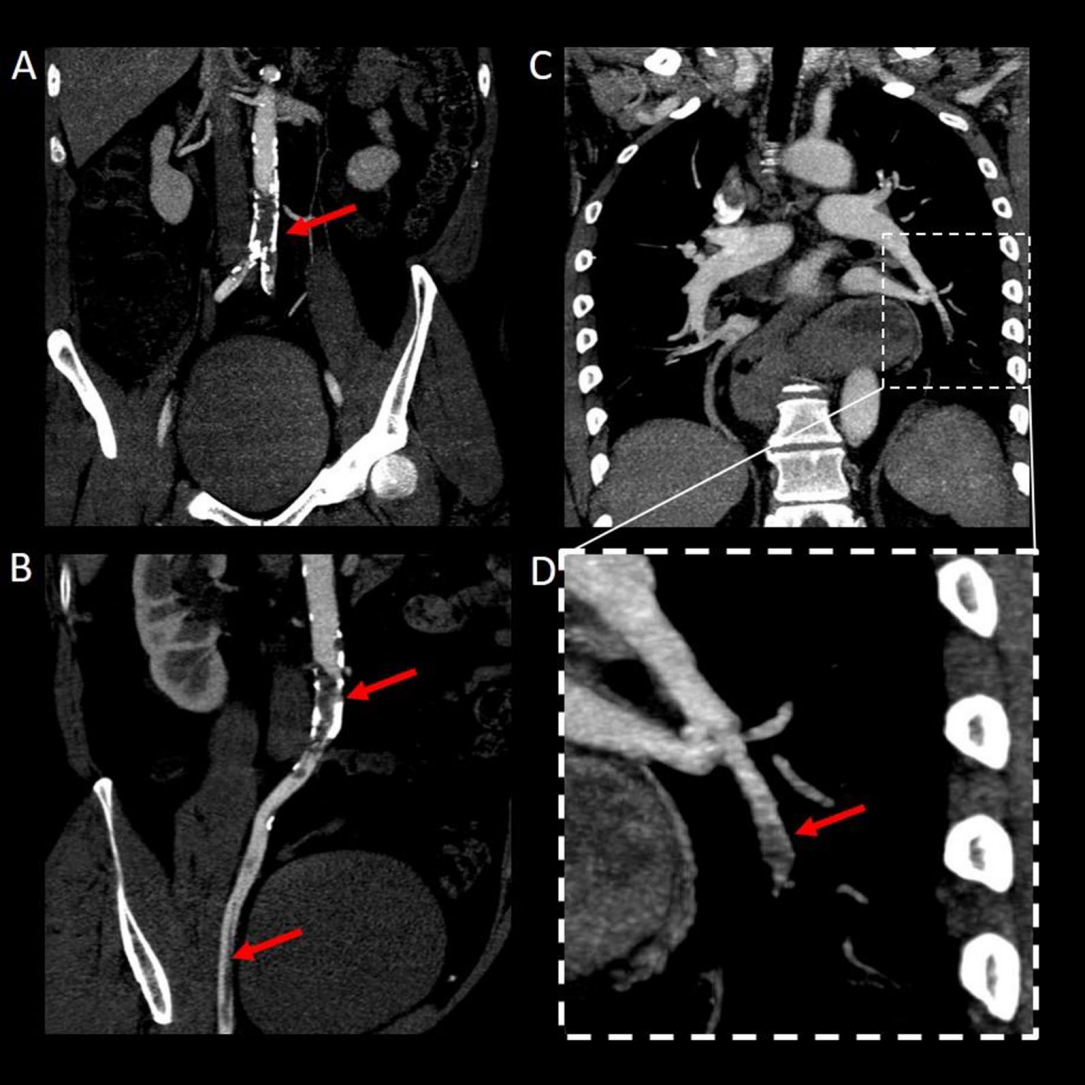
a Coronal and b curved multiplanar reconstructions of CT-A (in 5 mm maximum intensity projection) display subtotal thrombotic occlusion of infrarenal aorta and extension of thrombus into both common iliac arteries and right external iliac artery. c Coronal multiplanar reconstruction of CT-A (5 mm maximum intensity projection) and d magnified image show segmental pulmonary artery embolism
